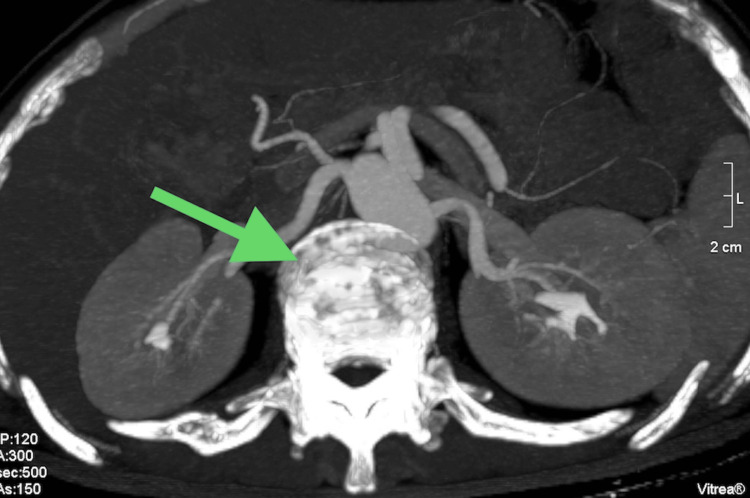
A computed tomography angiography of the abdomen (transverse plane); the arrow indicates a lytic lesion in the spine
